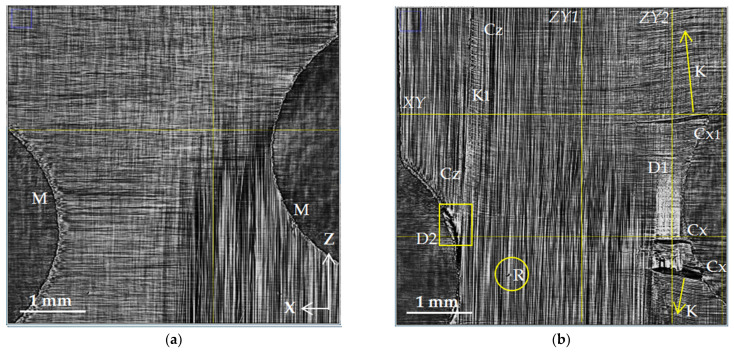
Microstructure of cross-ply CFR plastic at the interface of adjacent [90/0] layers: (a) initial state, 38 MPa; (b) loaded state, 865 MPa; M—mechanical damage after cutting; Cx—transverse crack spreading along the X-axis; K, K_1_—kinking of fibers; D—interlayer delamination; R—fiber rupture; XY, YZ1, and YZ2—positions of the orthogonal X-ray sections presented in Figure 8 and Figure 9.
